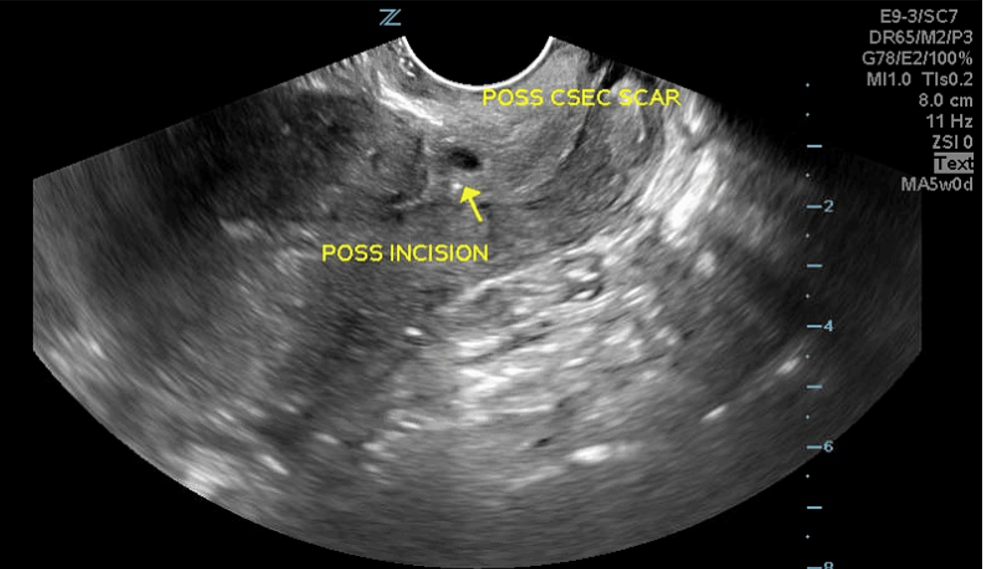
Transvaginal ultrasound (TVUS) showing gestational sac at the level of previous CS scar in the lower uterine segment
